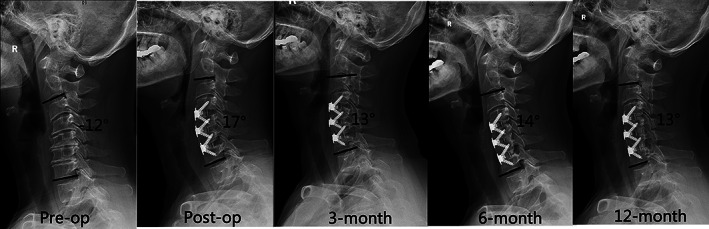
The follow‐up radiograph of patient employing intraoperative posture‐adjustment apparatus (IPAA). C7 slope (CL) increased from pre‐operative −12° to post‐operative 17°. Although the CL suffered a little decrease to 13° at 3‐month follow‐up, correction of CL was well maintained at 12‐month follow‐up.
